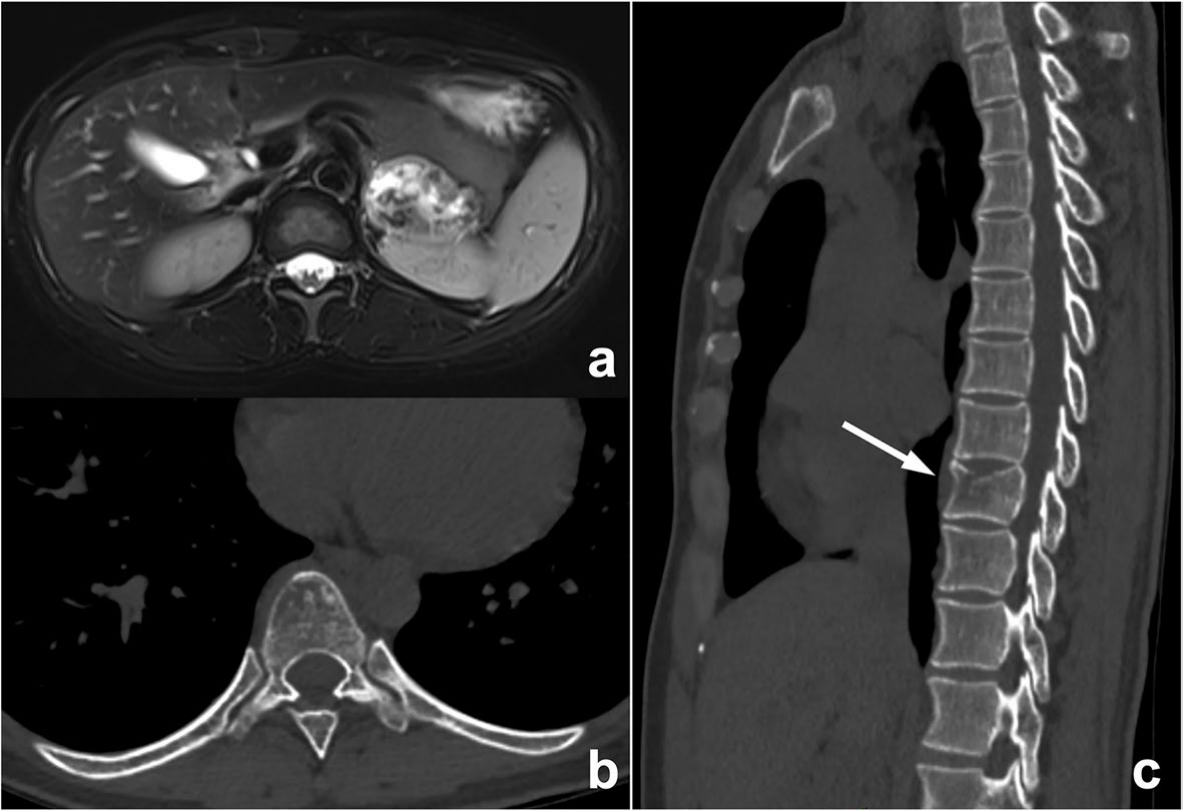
An abnormal signal mass of the left adrenal gland presented by abdominal MRI in a 39-year-old woman. In the routine preoperative chest CT scan (b, c), bone metastasis (arrow) was missed because radiologists mainly observed the pulmonary and mediastinal window but ignored the bone window. The diagnosis of postoperative pathology was left adrenocortical adenocarcinoma
